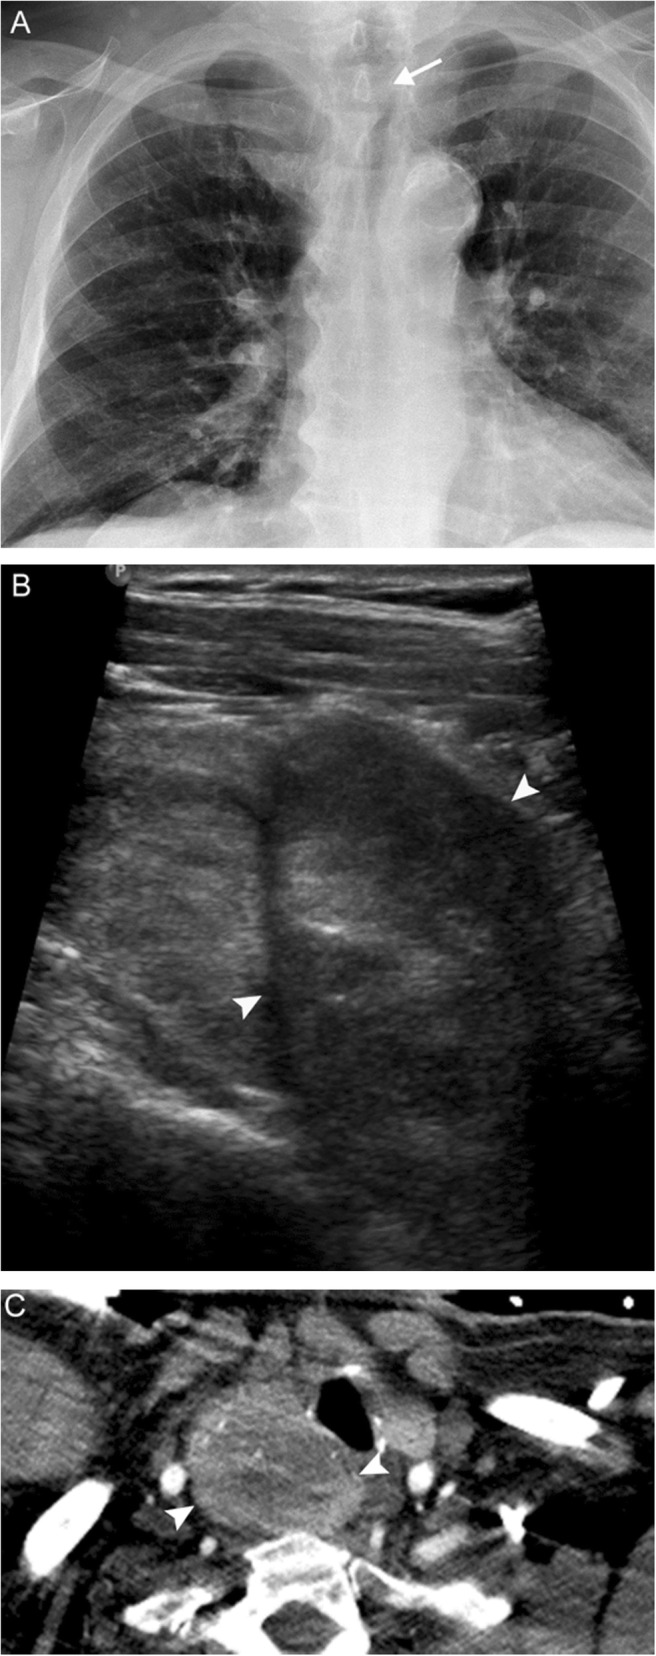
Thyroid carcinoma. A 78-year-old man presenting with persistent cough, hoarse voice, and dyspnea. Chest radiograph (a) reveals a right paratracheal mass indenting the subglottic trachea (white arrow). Subsequent neck US (b) and chest CTA (c) reveal a solid heterogenous mass with calcifications (white arrowheads), which narrows but does not invade the glottic/subglottic airway. Fine needle aspiration revealed follicular thyroid carcinoma
